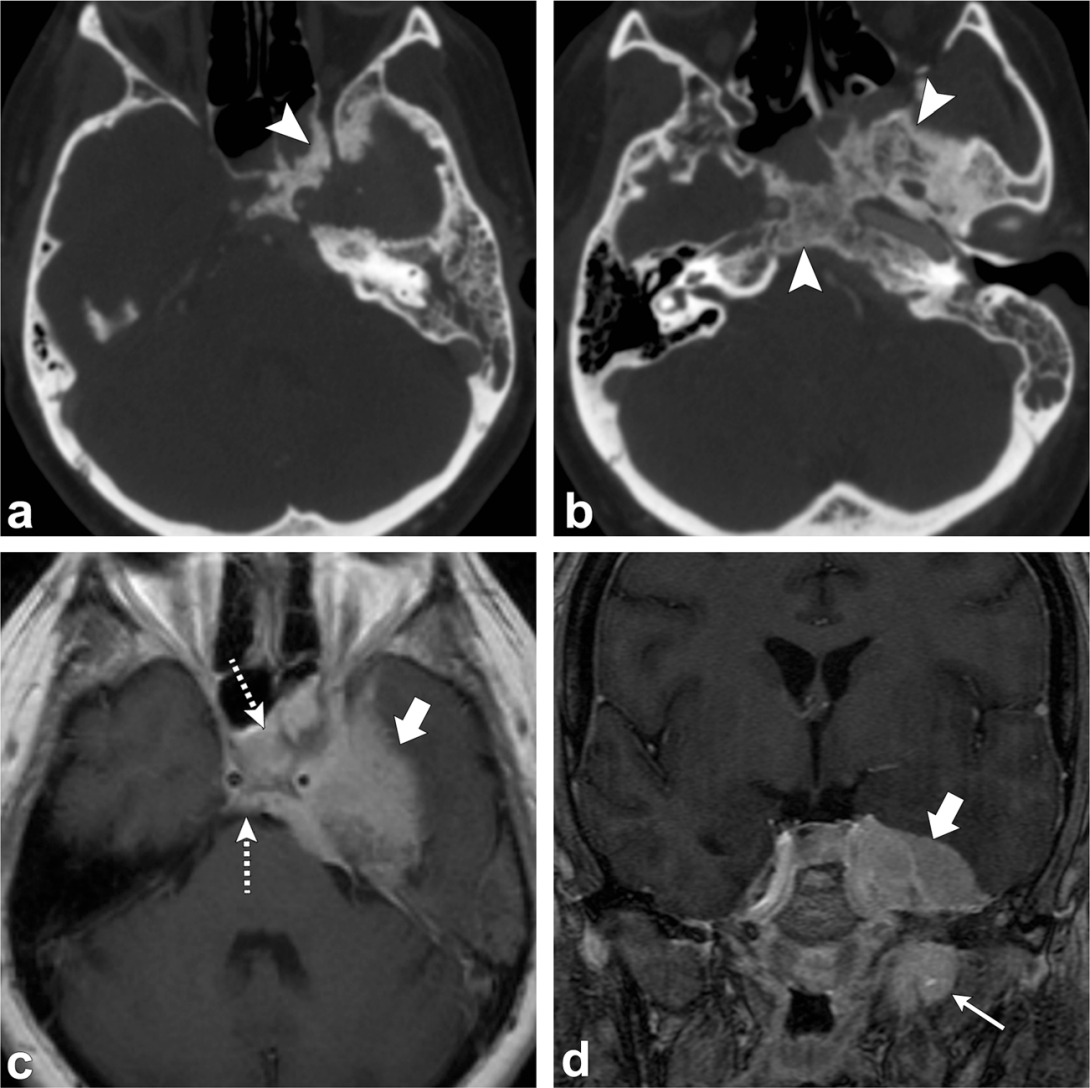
Intradural meningioma. Axial head CT images (a, b) show hyperostosis of the left sphenoid bone, clivus and petrous portion of the left temporal bone (*arrowheads*). Axial (c) and coronal (d) post-contrast T1-weighted images show a large enhancing lesion along the left sphenoid wing (*thick arrows*) extending along the clivus and sella (*dashed arrows*), as well as left middle cranial fossa (*short, thick arrow*); there is also extension outside of the calvarium (*thin arrow*)
